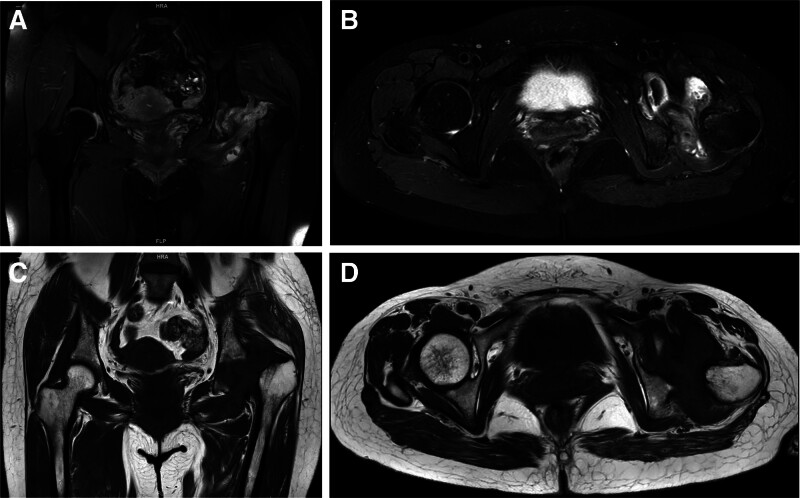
(A–D) Preoperative nuclear magnetic resonance imaging of the hip: (A) Preoperative anteroposterior nuclear magnetic resonance imaging demonstrating the normal structure of the left femoral head and femoral neck disappeared, and a small residual bony signal shadow could be seen. (B) Preoperative horizontal nuclear magnetic resonance imaging demonstrating the corresponding part of the synovial membrane was thickened, the bursa was swollen, and showed flaky FS-PDWI high signal, and a small liquid signal could be seen in the joint space. (C) Preoperative anteroposterior nuclear magnetic resonance imaging demonstrating the corresponding part of the left femur was shifted outwardly and superiorly. (D) Preoperative horizontal nuclear magnetic resonance imaging demonstrating the adjacent femoral end and acetabulum could be seen with flaky FS-TPD2WI high signal.
